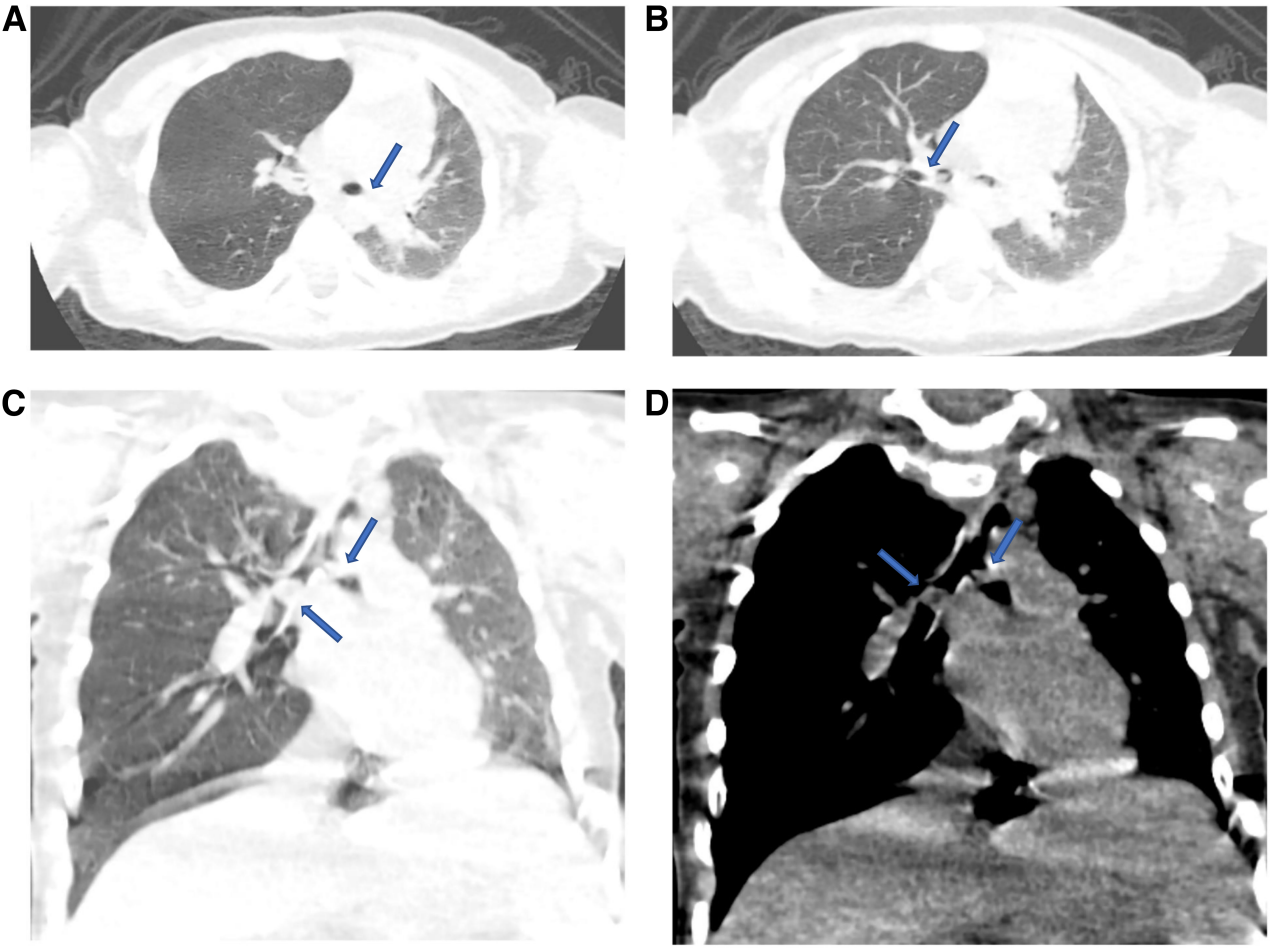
The location of bilateral TFB in patient were showed using the CT plain scan. (A,B) Bilateral TFB were shown from pulmonary window; (C,D) bilateral TFB were shown after three-dimensional reconstruction. TFB, tracheobronchial foreign body.
